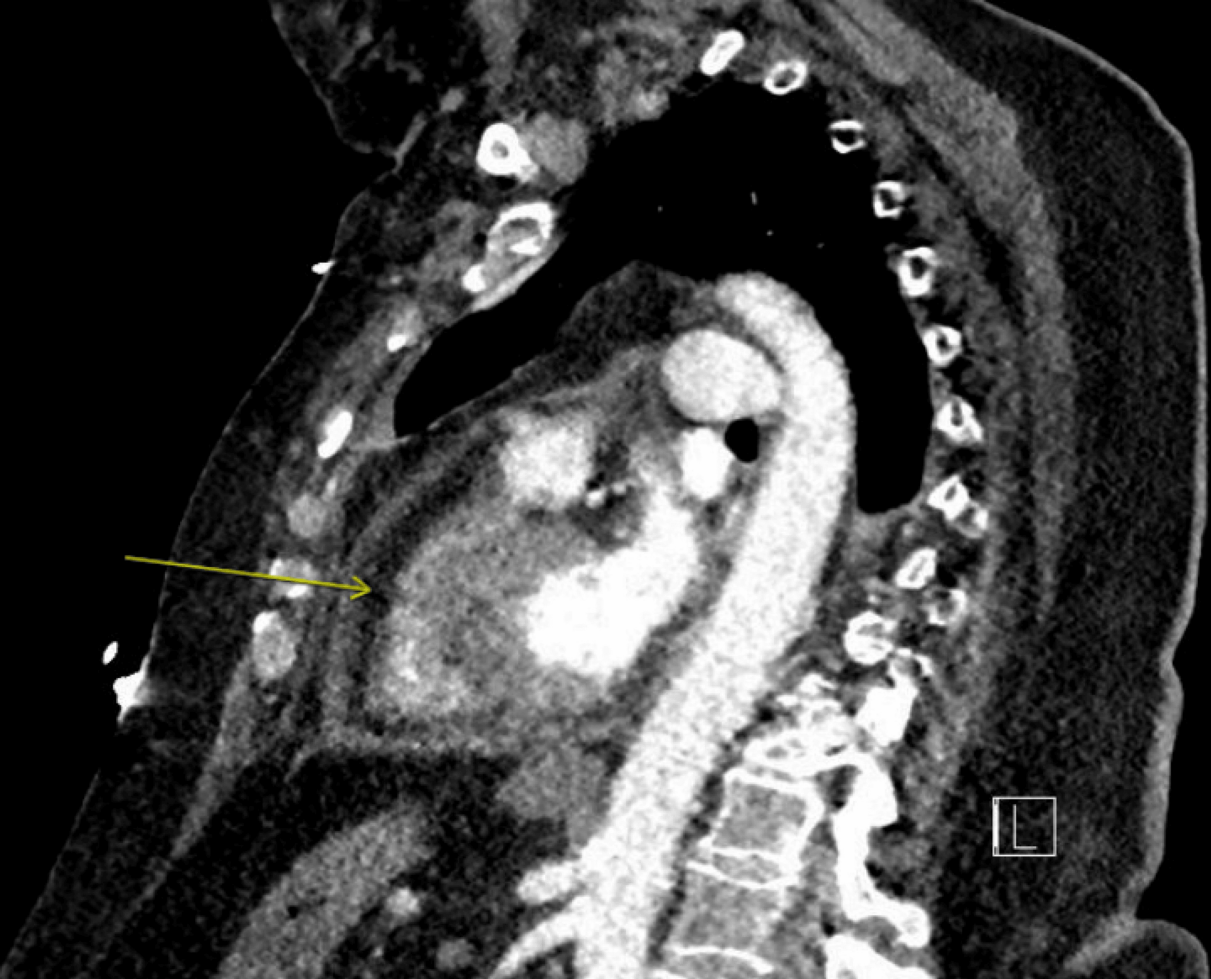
CT of the chest (sagittal cross-section) showing a moderate sized pericardial effusion (arrow)
